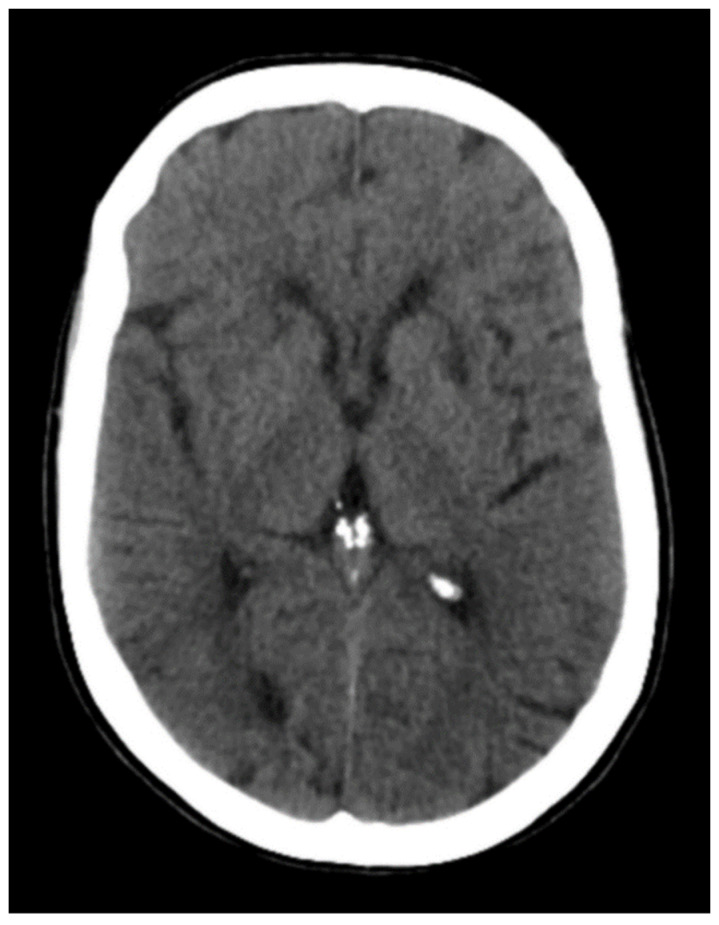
No intra- or extra-axial bleeding signs of acute ischemia (ASPECTS 10) or occupying lesions were observed in the simple cranial CT.
